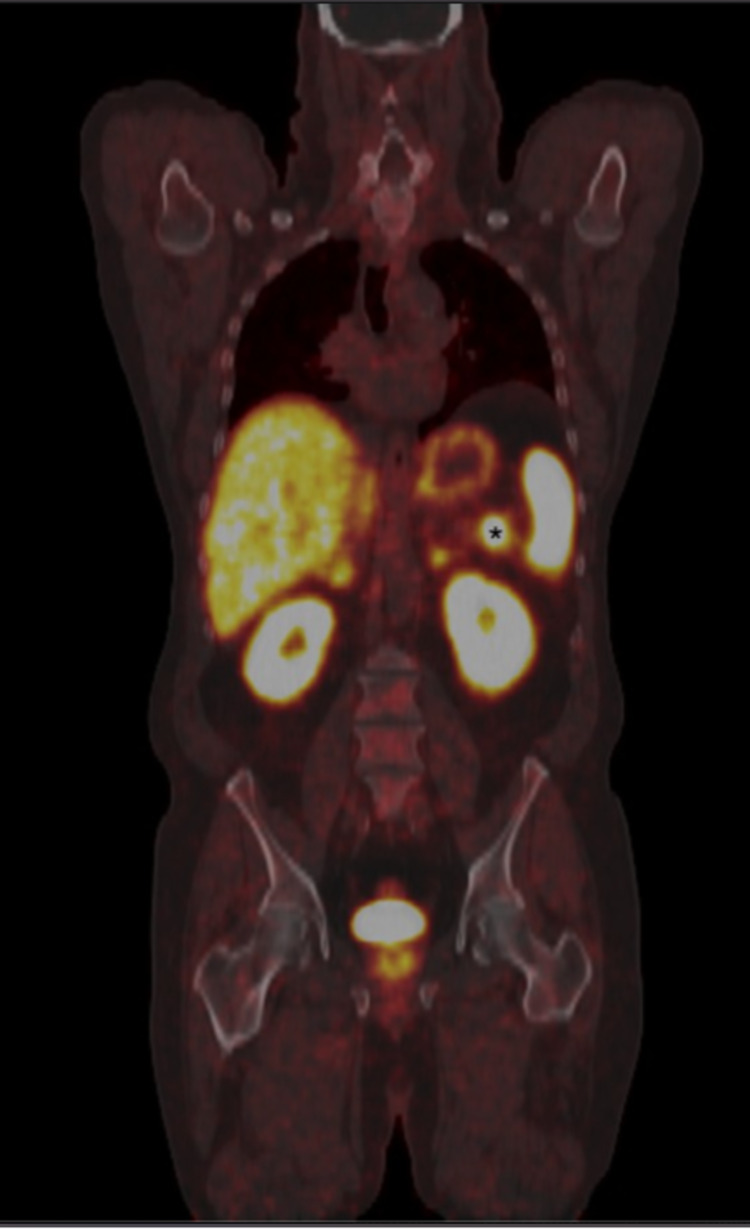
PET-CT NETSPOT, coronal view, with intrapancreatic tumor. Intrapancreatic tumor marked with (*) PET: Positron emission tomography
